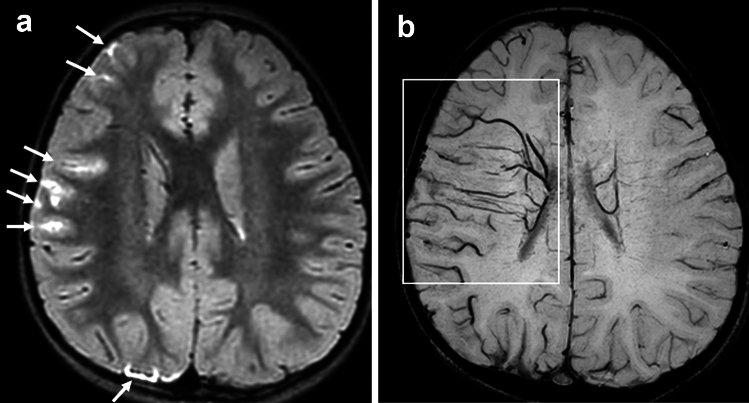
A 3-year-old girl with Sturge–Weber syndrome. She had 5 seizures at age 1 year, but has been seizure-free since taking carbamazepine. Contrast-enhanced FLAIR shows strong enhancement of several sulci on the right side (a, arrows). SWI shows enlargement of the deep medullary and subependymal veins on the right side (b, square)
